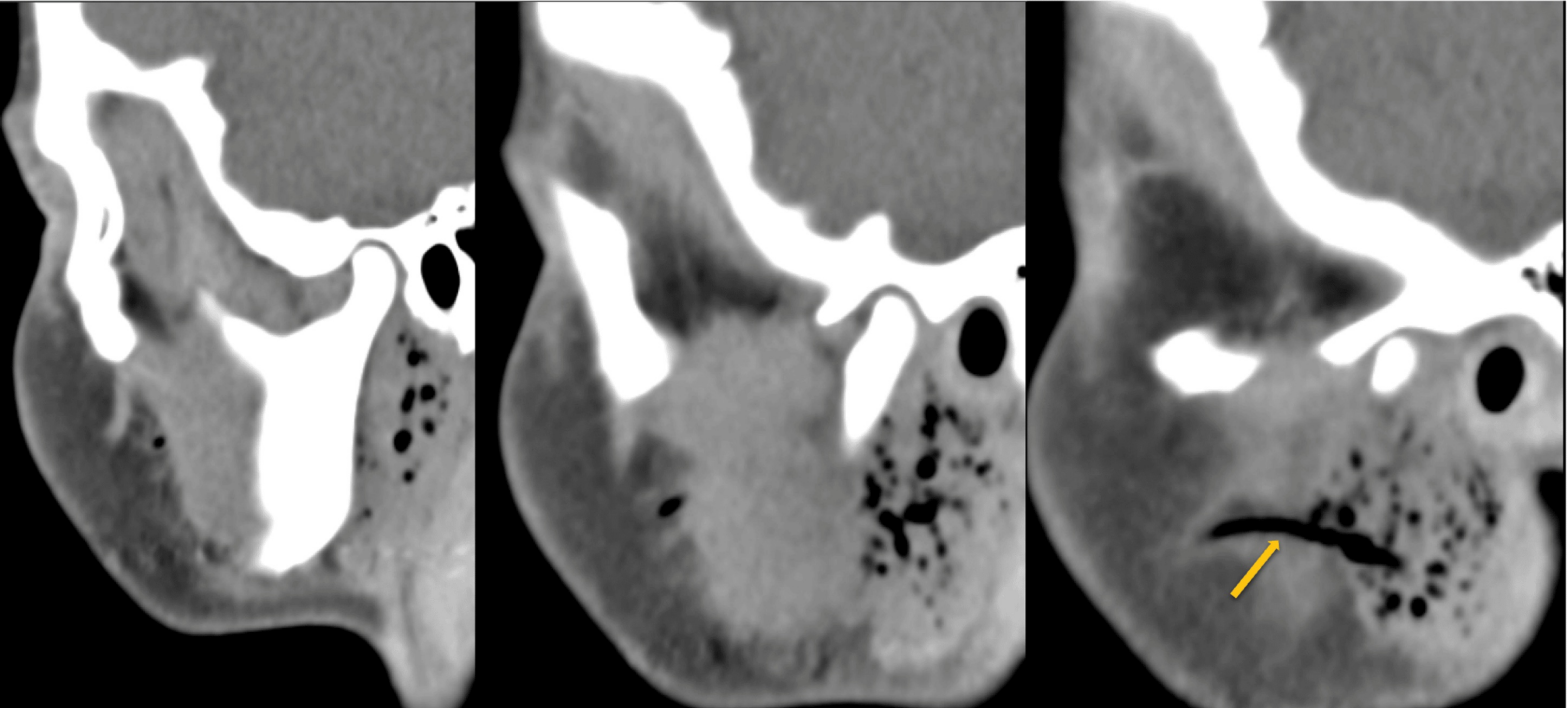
CT scan Sagittal CT sections demonstrate enlargement of the right parotid gland with multiple millimeter-sized intraglandular gas bubbles and no signs of inflammation. Gas is also observed within Stensen’s duct, extending to the level of the buccinator muscle (arrow).
